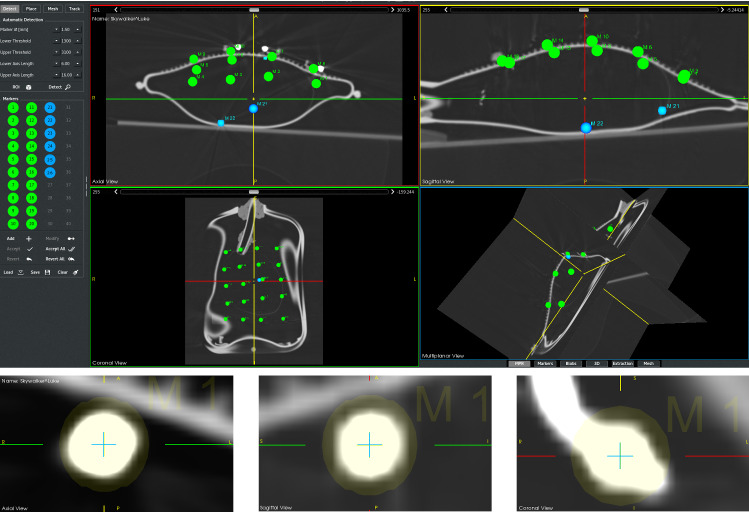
Top: 3D positions of the surface and target marker centroids were detected and localized automatically and managed in a fiducial list for each 4D-CT phase, respectively. 20 green spheres on the images show the localized and accepted surface markers and blues, candidate target markers to be accepted. Bottom: Enlarged CT views of detected RS ball. It is clearly visible that the crosshair (indicates the localization position) is located in the center of the ball
